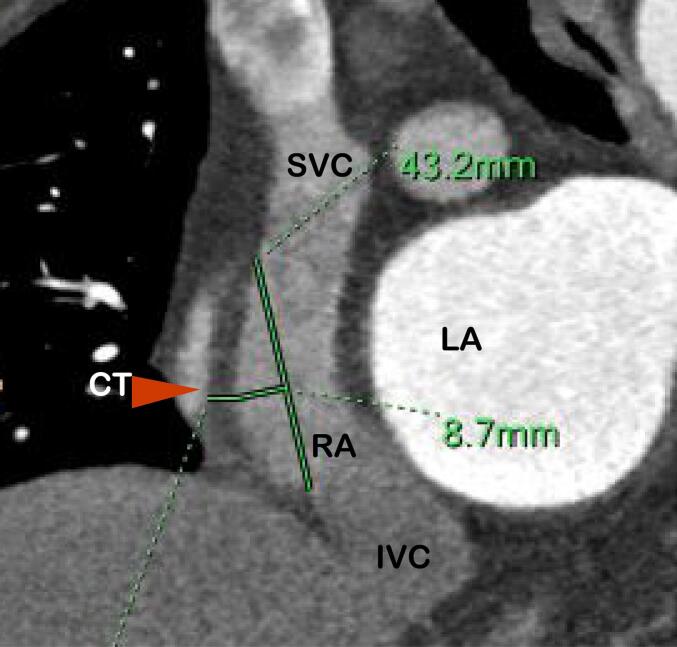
Measurement of the crista terminalis (CT)thickness: The optimal coronal image to display the crista terminalis was determined, which extends from the superior vena cava to the inferior vena cava. A line was drawn from the right margin of the superior vena cava (SVC) to the right margin of the inferior vena cava (IVC), and intersect this line with a perpendicular line passing through its midpoint. The thickness of the crista terminalis in this region was measured.
